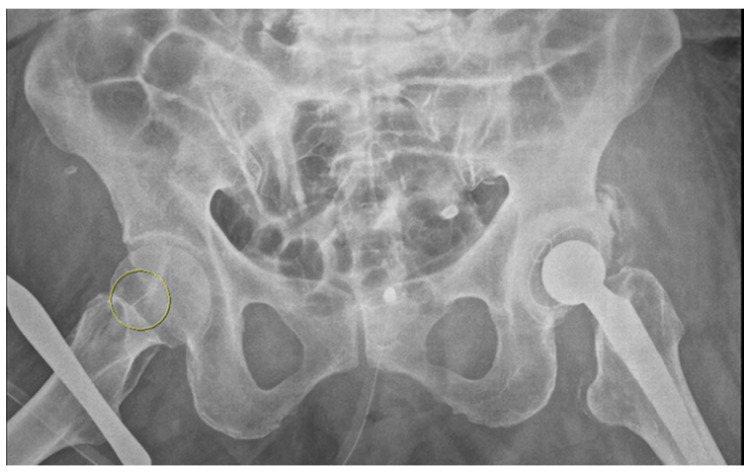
Radiography of pelvic bone. A needle fragment showed in the right groin (yellow circle).
